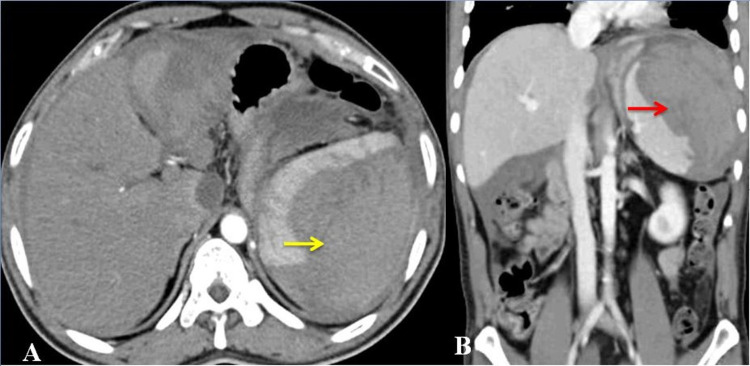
Computed tomography showing (A) arterial phase showing peri-splenic hematoma (yellow arrow) (axial view) and (B) venous phase showing peri-splenic hematoma (red arrow) (coronal view).
